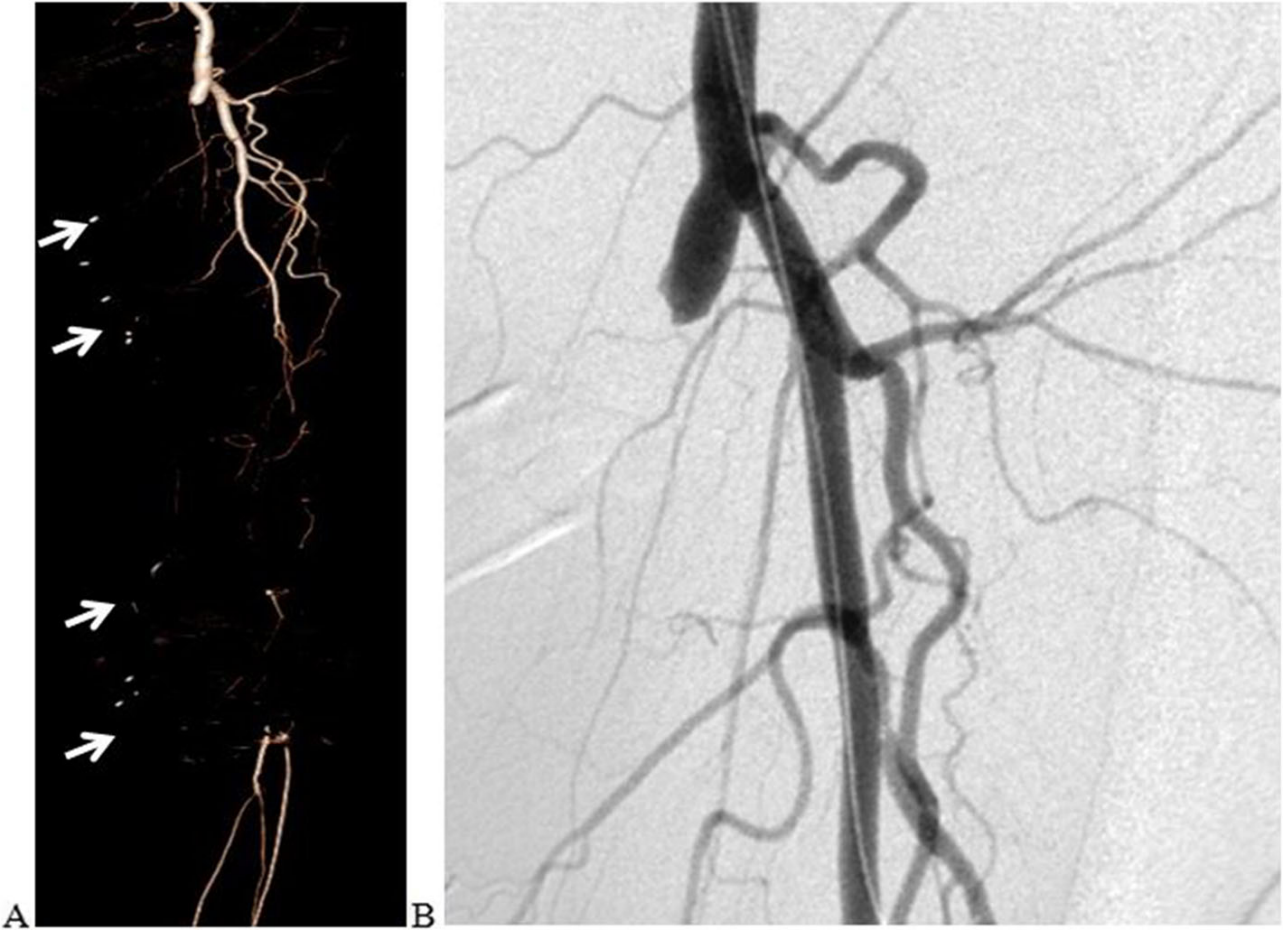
Fluoroscopic images (A) demonstrating percutaneous transluminal angioplasty of all portions of the left superficial femoral artery. Subsequent angiography (B) shows PTA failure, defined by a flow-limiting dissection, after which multiple stenting is performed (C). Digital subtraction angiography (D) demonstrates successful recanalization of the left superficial femoral artery. A computed tomography angiography with 3D reconstruction (E), performed at 12 months follow-up, confirms patency of the native left superficial femoral artery
